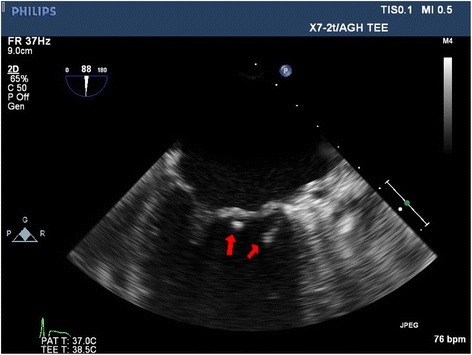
Transesophageal echocardiogram showed two vegetations on the patient’s mitral valve. *Red arrows* point to the vegetations
